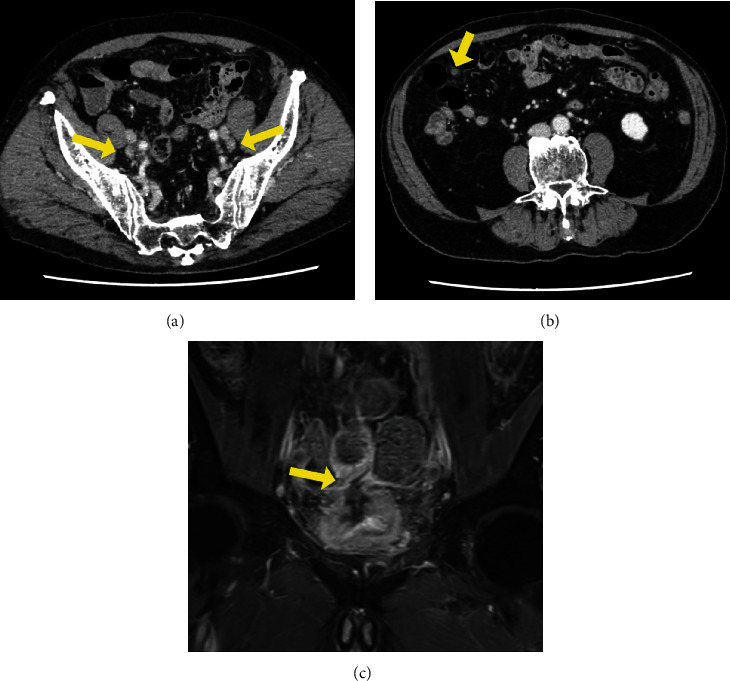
A contrast-enhanced abdominal computed tomography (CT) scan for staging showed bilateral iliac regional lymph node enlargement (a, arrow) and a small nodule in the peritoneum (b, arrow). (c) A T1-weighted TSE magnetic resonance imaging scan for preoperative local evaluation showed that the soft-tissue mass on the bladder wall extended to the small intestine (arrow), suggesting local invasion.
